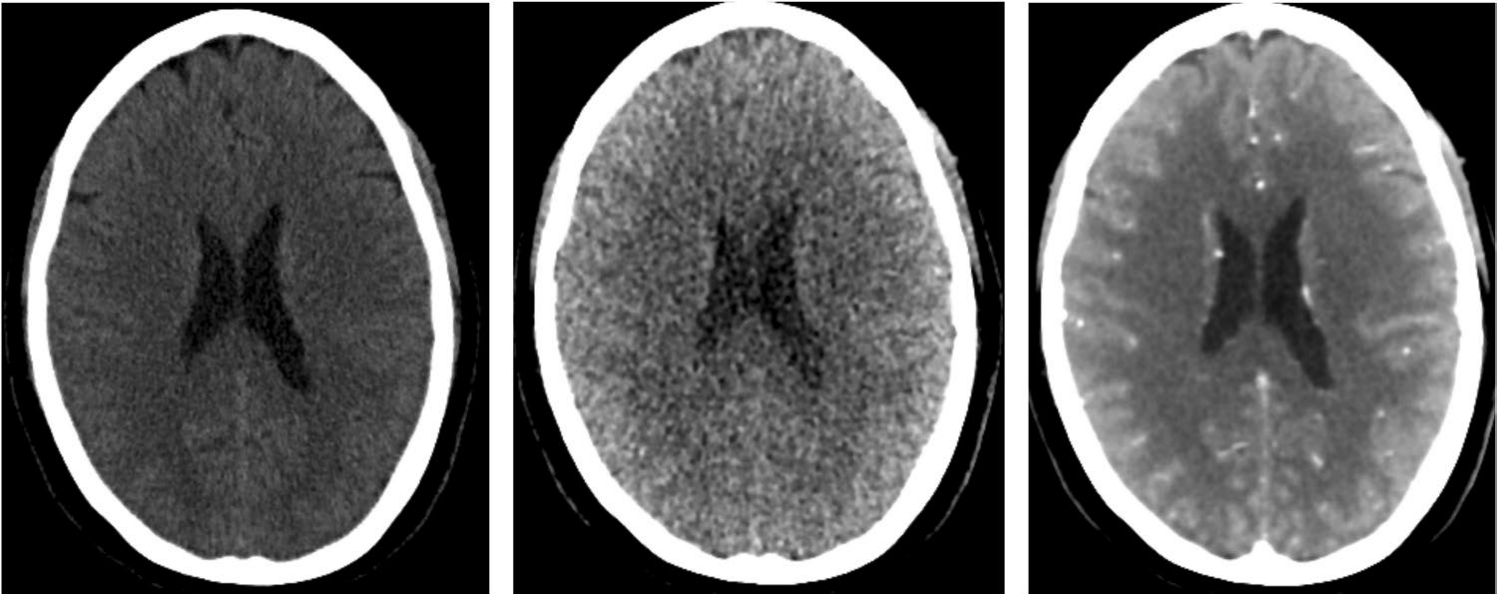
Axial slice at the level of the ventricles of a non-contrast CT acquired with 220 mAs exposure (left), the first time point of the 4D CT at 200 mAs, and the weighted temporal average of the 4D CT at a total exposure of 2250 mAs. All images are visualized with the same window level and width of 60 and 120 HU.
